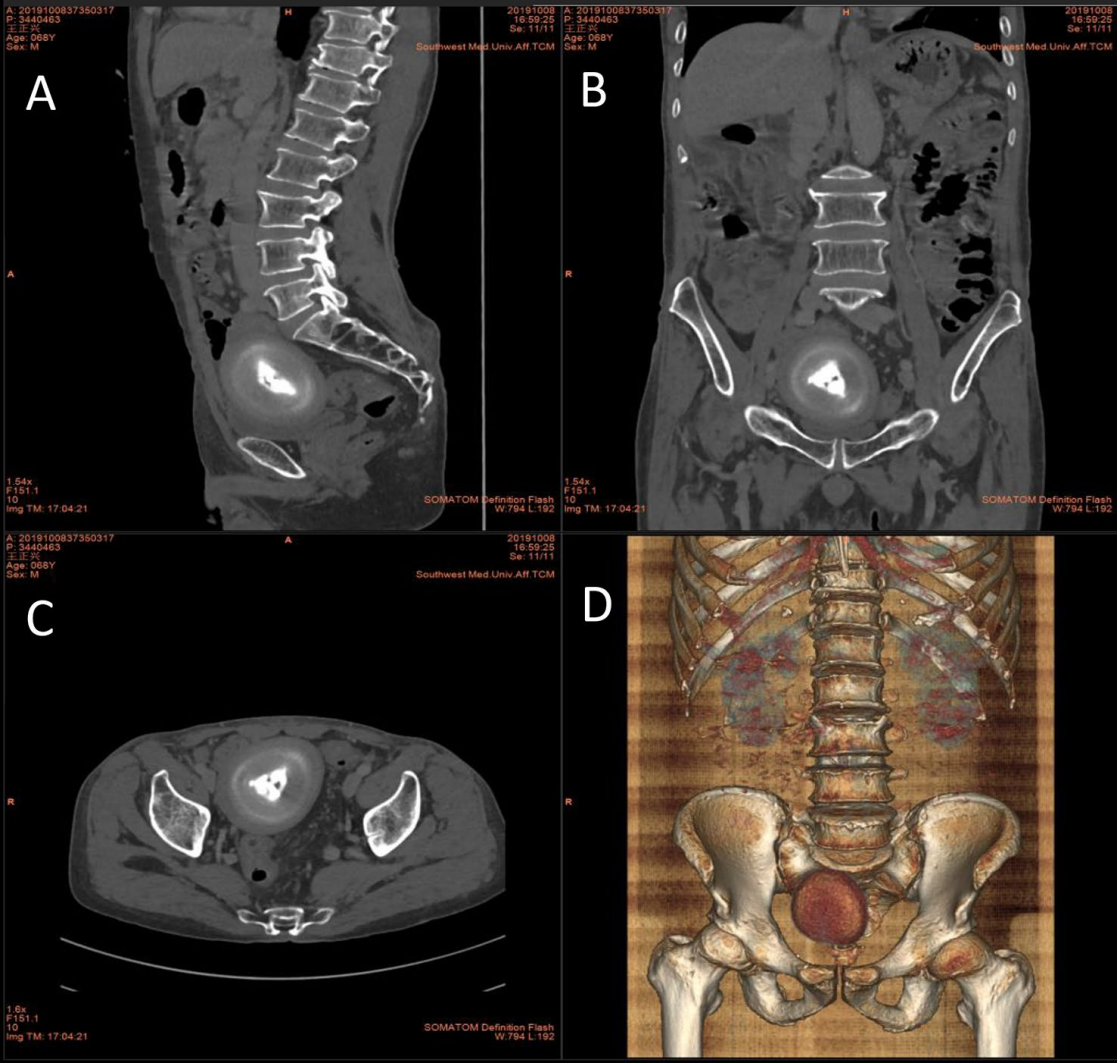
CT scan: A rounded, mixed-density mass was founded in the pelvis, measuring approximately 11.5 × 8.6 × 7.4 cm. A: sagittal; B: coronal; C: transverse; D: 3D image reconstruction
